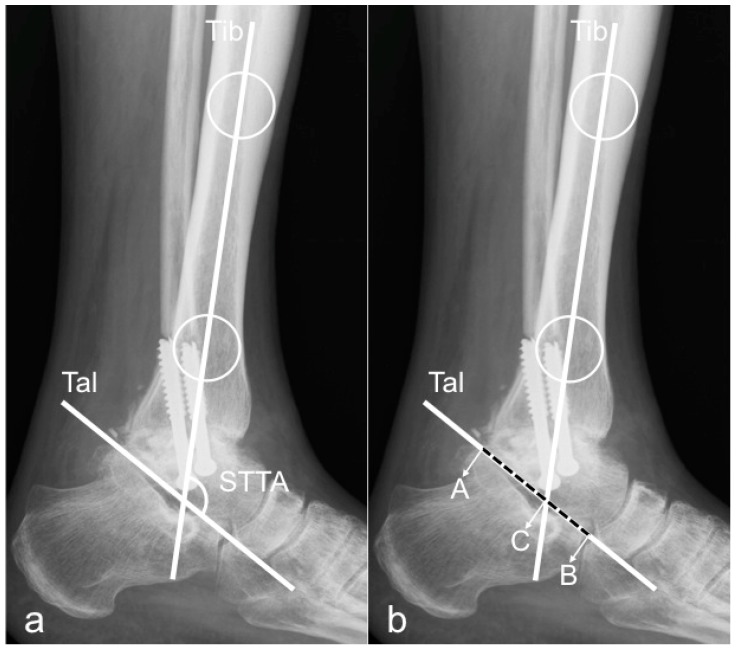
Method 1 for STTA and mTT-ratio measurement. Lateral ankle radiograph demonstrating Method 1: Tib = longitudinal axis of the tibia, created by connecting two points in the middle of the proximal and distal tibial shaft. Tal = axis of the talus, defined by a line drawn from the inferior aspect of the posterior tubercle of the talus to the most inferior aspect of the talar neck. Figure (a) depicts measurement of the sagittal tibio-talar angle (STTA). Figure (b) shows measurement of the modified tibio-talar (mT-T) ratio.
